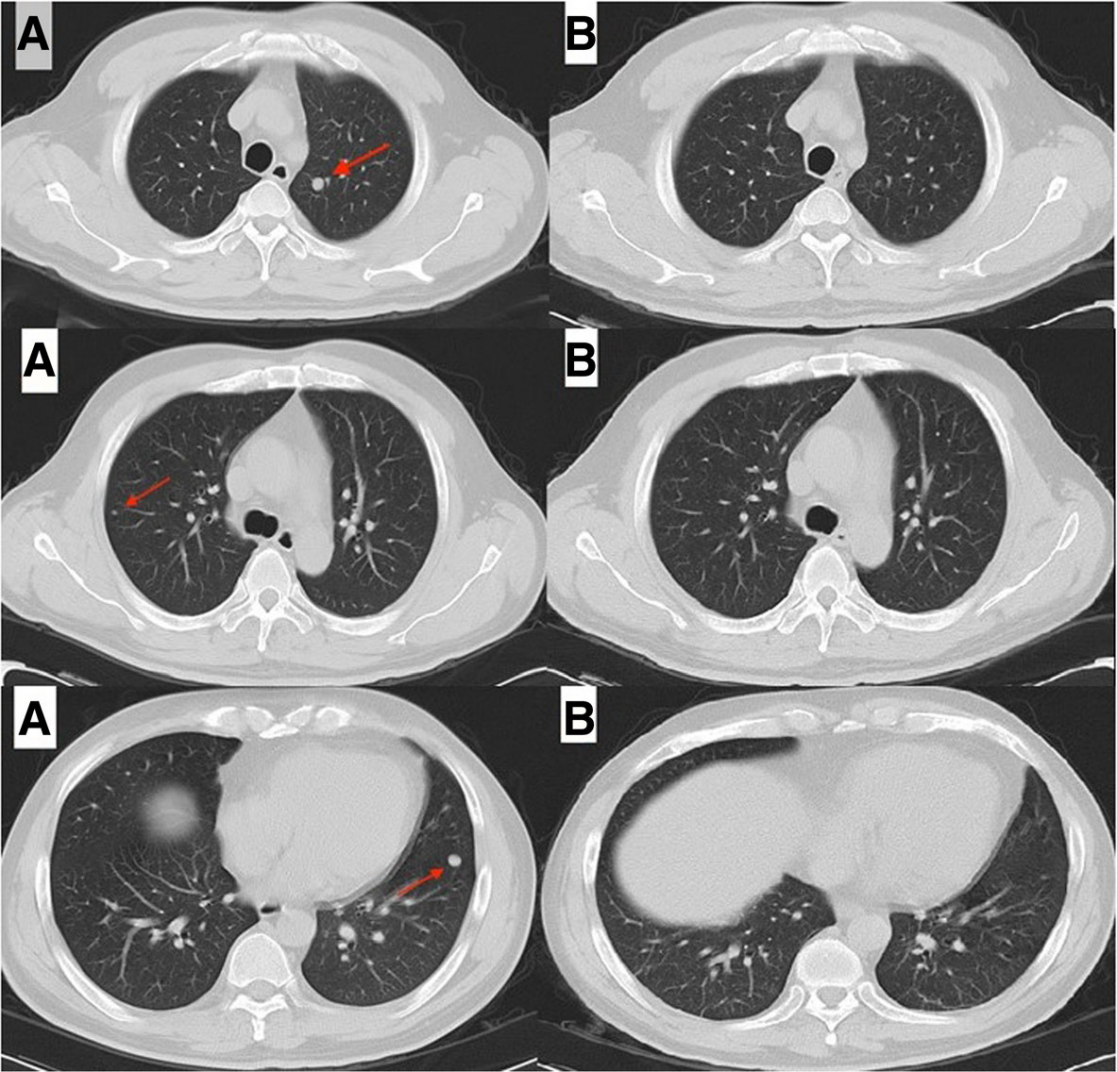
Chest CT images. (a) Follow-up chest CT images taken 3 months after surgery on January 10, 2017 demonstrated three new lesions (arrows) in the bilateral pulmonary region, before treatment with cizotinib. b Follow-up chest CT images taken on February 20, 2017 at 4 weeks after the initiation of oral crizotinib administration indicated improvement
